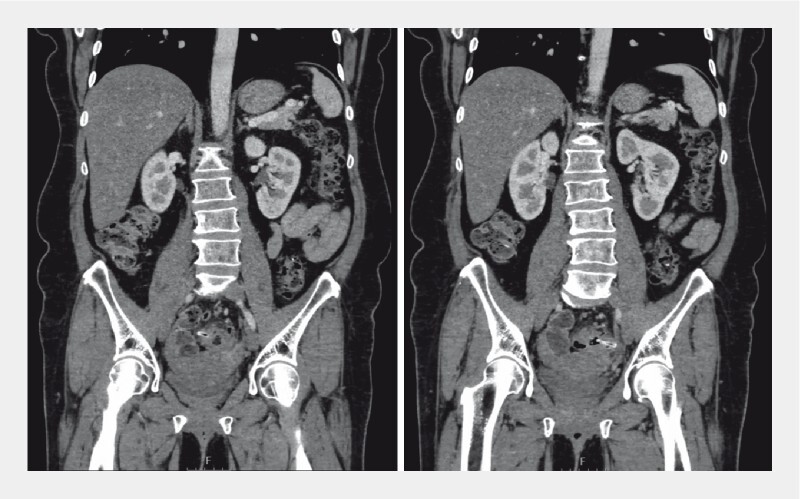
Coronal abdominal computed tomography showing the chicken bone measuring 55 mm in length located in the sigmoid colon, transversely oriented to the colon axis, without signs of perforation.
